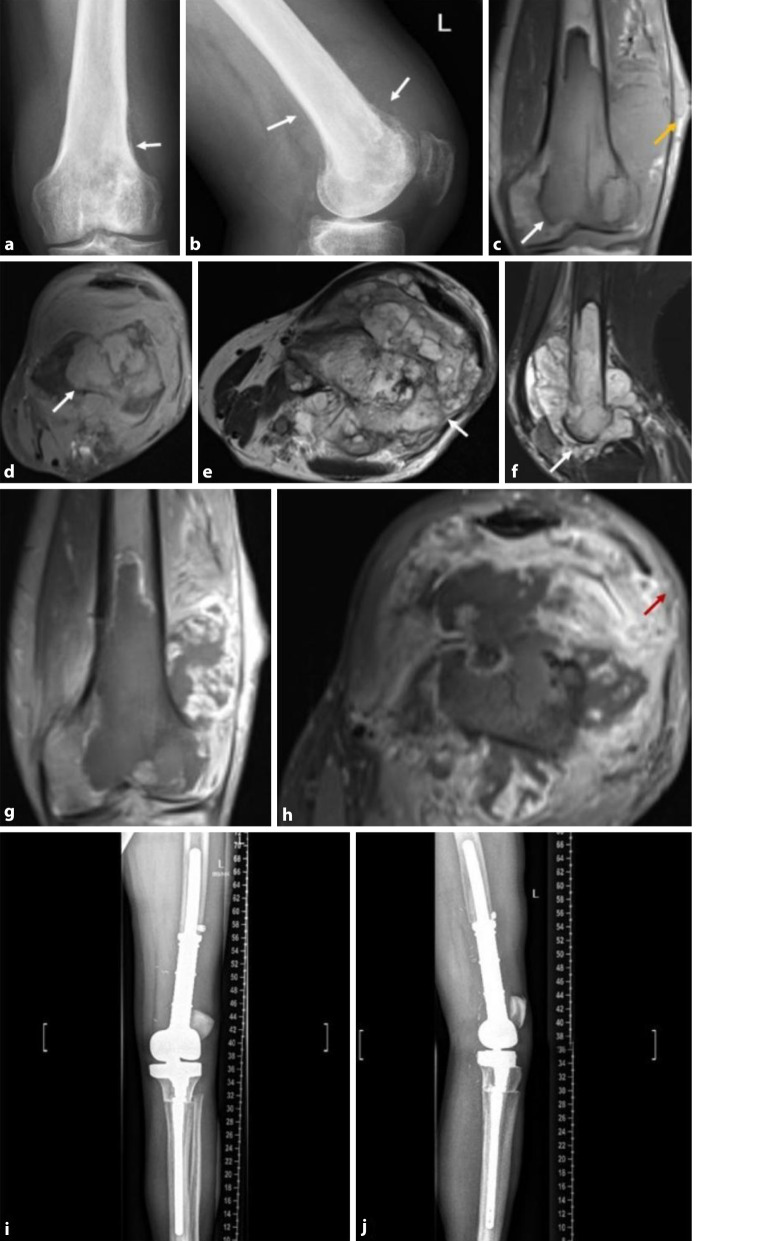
Case 2. a, b Anteroposterior (AP) and lateral radiographs of the distal femur showing a heterogeneous bone structure with aggressive periosteal reaction along the distal medial metadiaphysis (a, arrow) and pressure erosion. On MRI, the lesion shows hyperintense appearance on T1-weighted images (c, d) and heterogeneous hyperintense signal on T2-weighted images with circumferential cortical destruction (e) and joint infiltration (f). After intravenous contrast administration (g, h) typical nodular enhancement of cartilaginous tumor tissue is shown with biopsy tract (h, red arrow) on the medial side. i, j Postoperative radiographs in AP (i) and lateral view (j) after wide resection with modular tumor knee endoprosthesis
